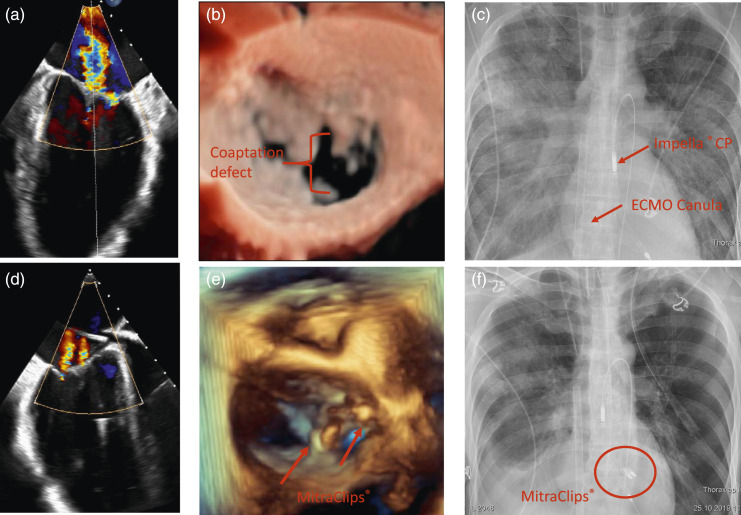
Transesophageal echocardiography of the mitral valve
